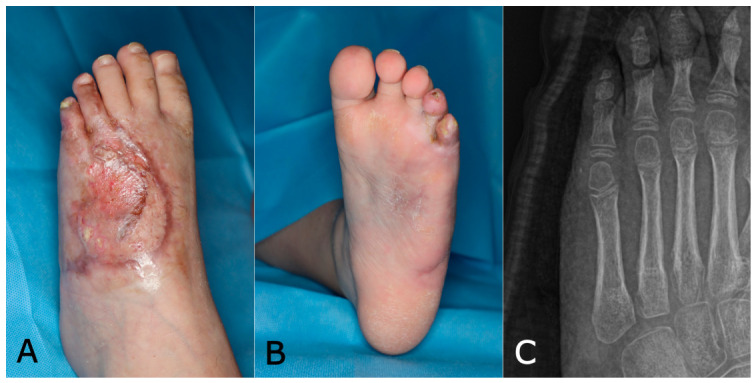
Clinical photographs and radiograph at follow-up. (A,B) Follow-up clinical photographs demonstrating preserved flap viability and stable wound healing, without significant scar contracture or deformity. (C) Follow-up radiograph demonstrating satisfactory alignment of the phalanges.
